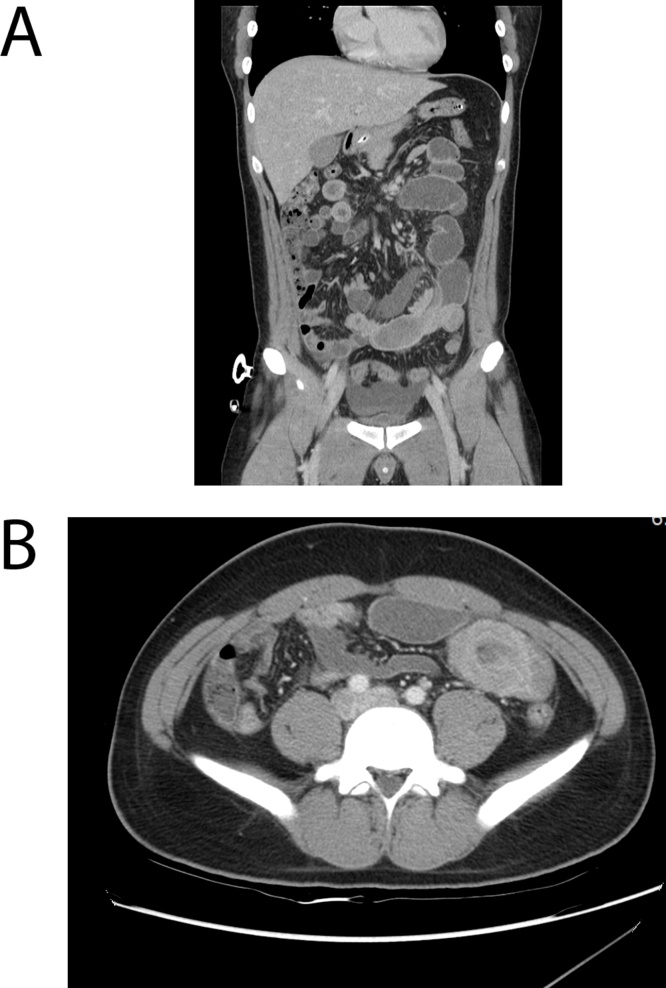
Computed tomography revealing intussusception. (A) coronal view; (B) axial view.
